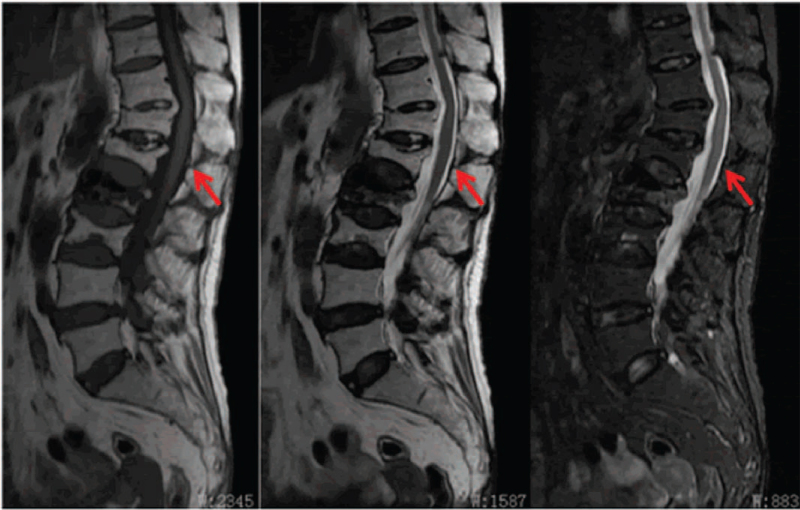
The MRI at 3-month follow-up revealed complete resolution of the spinal epidural hematoma (indicated by red arrow). MRI = magnetic resonance imaging.
